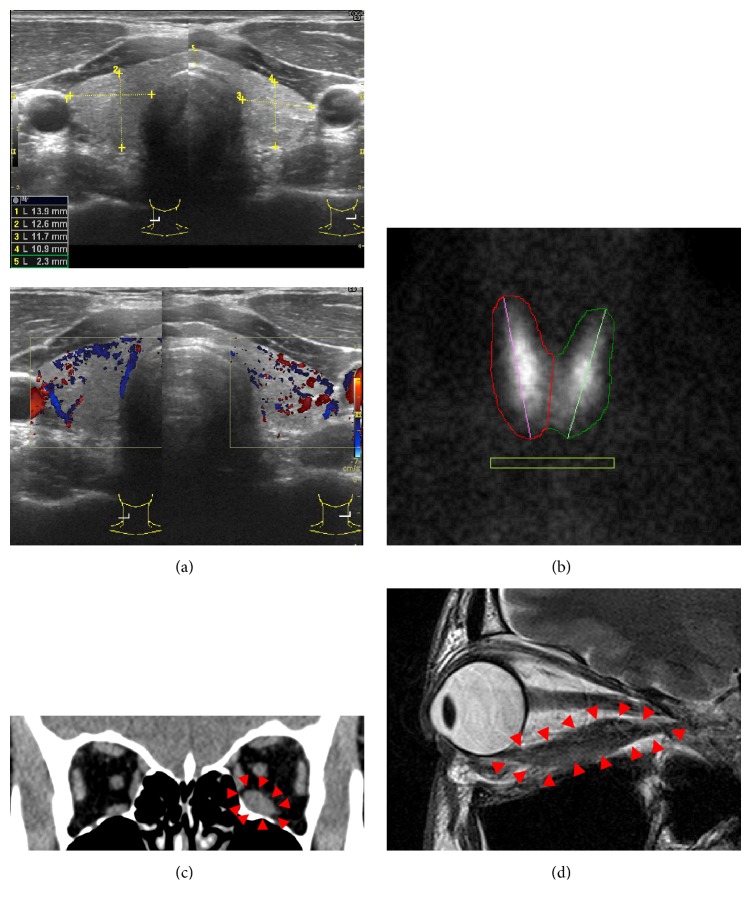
Imaging studies. (a) Ultrasonographic images of the thyroid showing normal-sized gland with slightly enhanced blood flow. (b) ^99m^Tc scintigraphy exhibiting an uptake within the normal range. (c) CT scan image showing enlarged inferior rectus muscle of the left eye. (d) Fat-suppression T2-weighted MRI image of the left eye showing inferior rectus muscle swelling with slightly high intensity signal of the muscle body.
